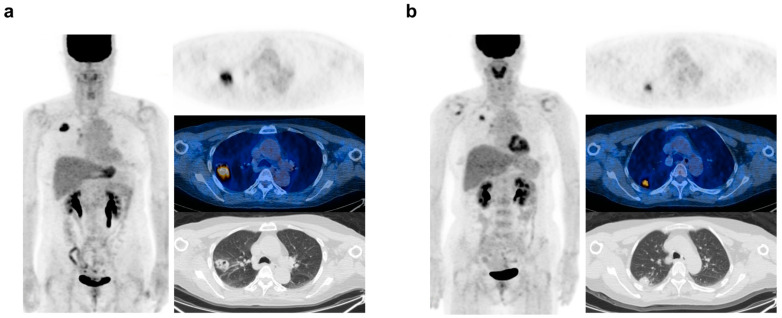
Representative PET/CT images of NSCLC patients following right upper lobectomy for adenocarcinoma. (a) Maximum intensity projection (left), transaxial fusion PET/CT (right middle), and CT (right bottom) images of a 74-year-old female with a high FDG-based risk score of 0.171. The patient’s pathologic tumor stage was I [T2 (tumor size 3.5 cm) N0 (no metastasis after mediastinal lymph node dissection)], but she died 20 months after surgery due to recurrence. (b) Maximum intensity projection (left), transaxial fusion PET/CT (right middle), and CT (right bottom) images of 61-year-old female with a low FDG-based risk score (0.076). The patient’s pathologic tumor stage was III [T2 (tumor size 2.2 cm, but invaded the visceral pleural beyond the elastic layer) N2 (identified metastasis in right pulmonary hilar and lower paratracheal lymph nodes after mediastinal lymph node dissection)], but she was alive at the five-year follow-up.
